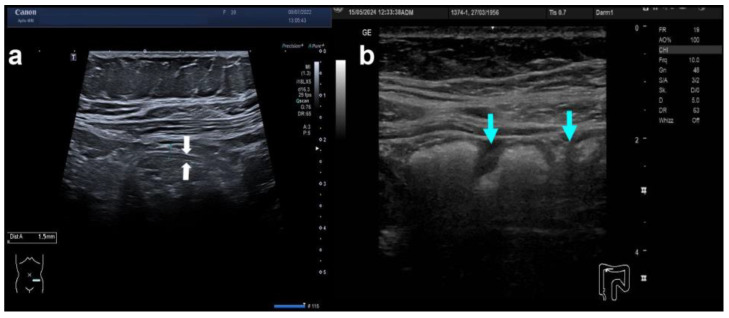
Intestinal ultrasound: normal colon. The transverse (a) and longitudinal (b) section of a normal colonic wall are shown. On the transverse section (a), the white arrows show the inner and outer margin with five layers in between (the white interface, the dark mucosa, the white submucosa, the dark muscularis propria, and the white serosa as the outer layer). On the longitudinal section (b), the normal haustration is indicated by the turquoise arrows.
